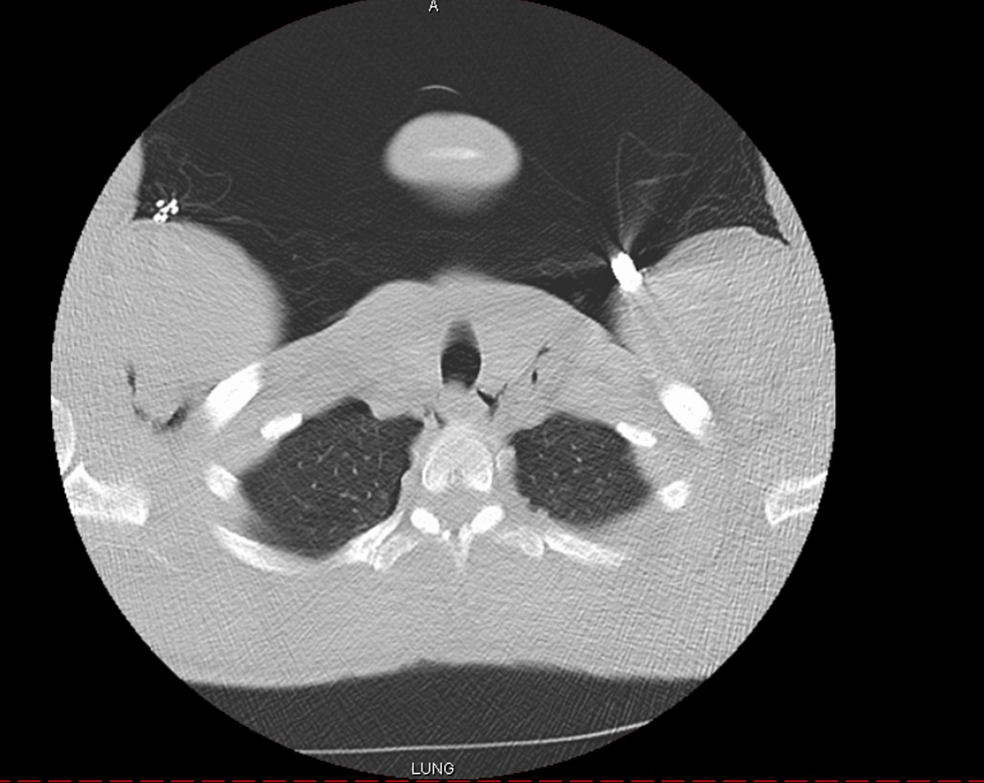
Axial computed tomography slice of the apical lungs highlighting the absence of pulmonary blebs.
